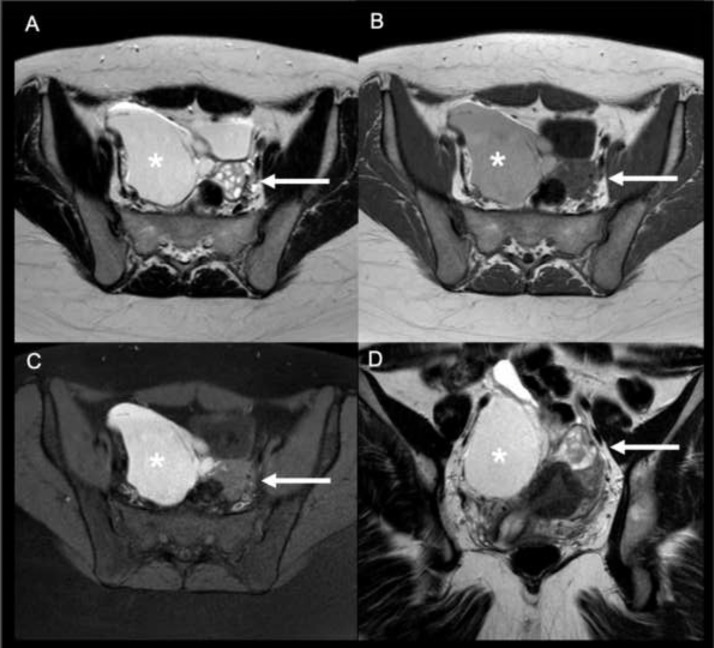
Axial T2-weighted TSE (A), axial T1-weighted TSE (B), axial T1-weighted SPIR (C) and coronal T2- weighted TSE (D) scans of the pelvis. The lesion (*) appears partially hyperintense on T1 and T2 weighted scans due to the proteinaceous-haemorrhagic content. Note the right ovary shifted to the left side (arrows).
